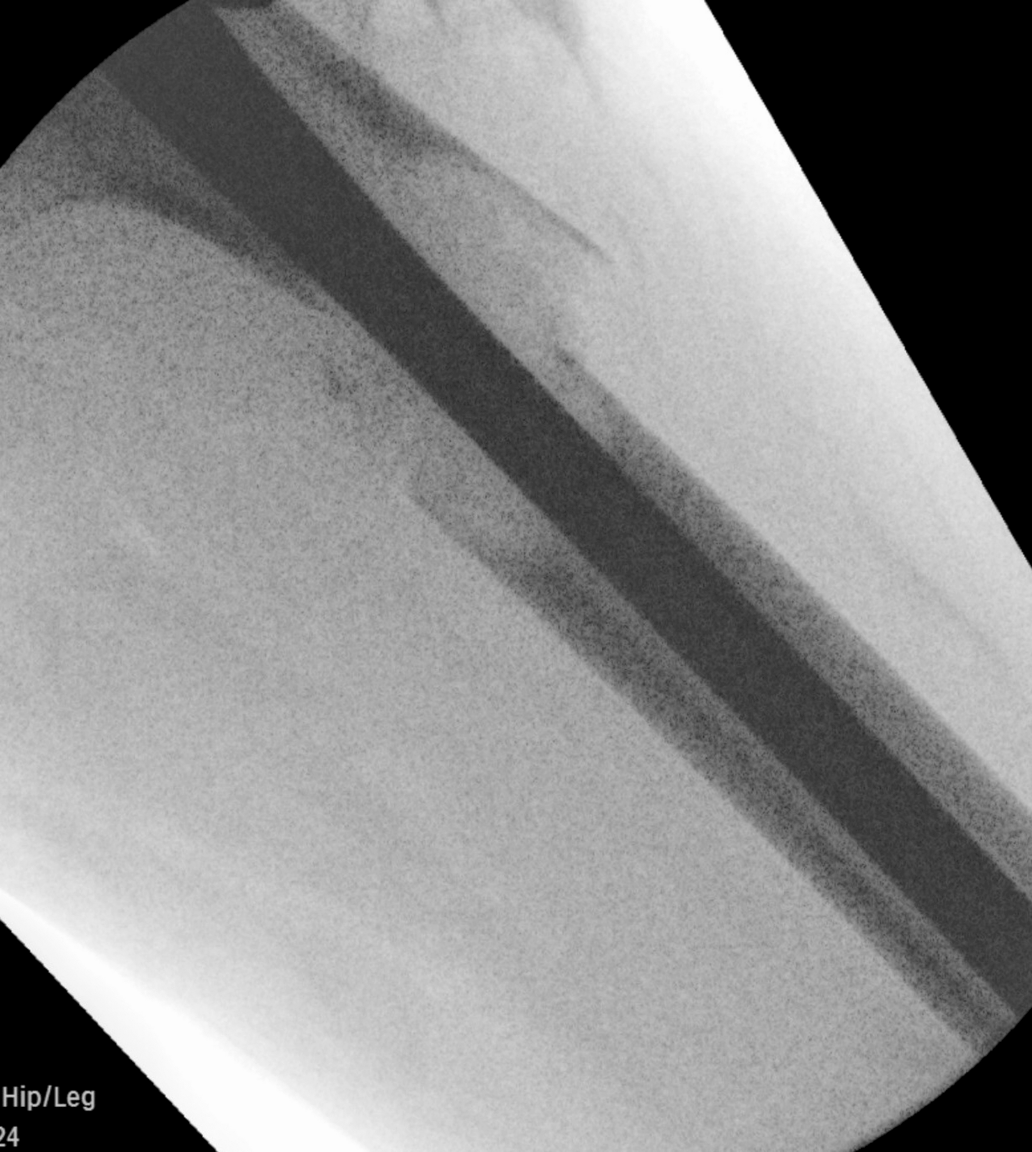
Intraoperative X-ray showing the long femoral gamma nail fixation of the left femoral shaft fracture
